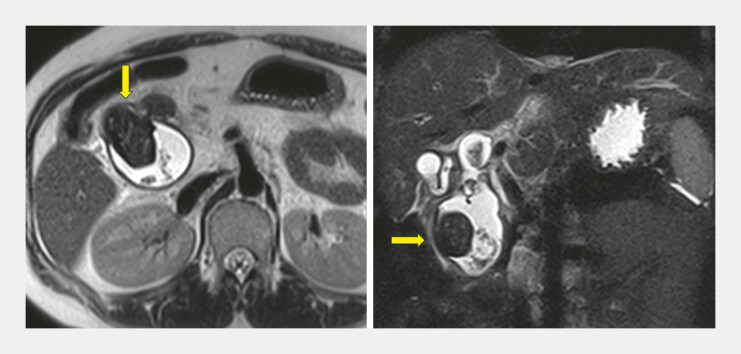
In an axial view, T2-weighted magnetic resonance imaging showed a large stone in the dilated common bile duct protruding into the duodenum (left). A coronal view revealed multiple stones in the dilated common bile duct (right).
